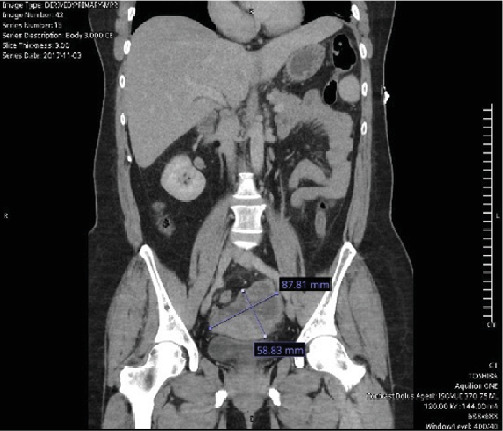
CT scan at time of diagnosis. Coronal image identifying 8.7 *cm* × 5.8 *cm* heterogeneous cystic lesion.
